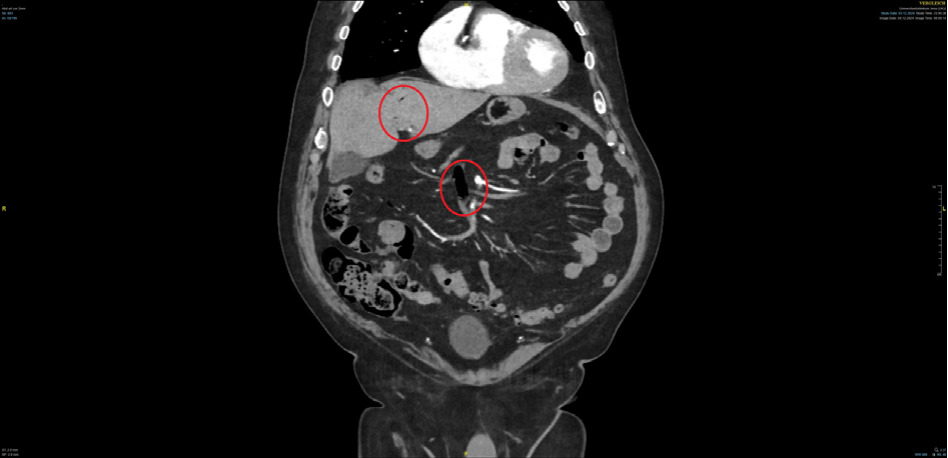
Frontal view of an abdominal angiographic computed tomography scan. Red circles mark gases in the venous system.
